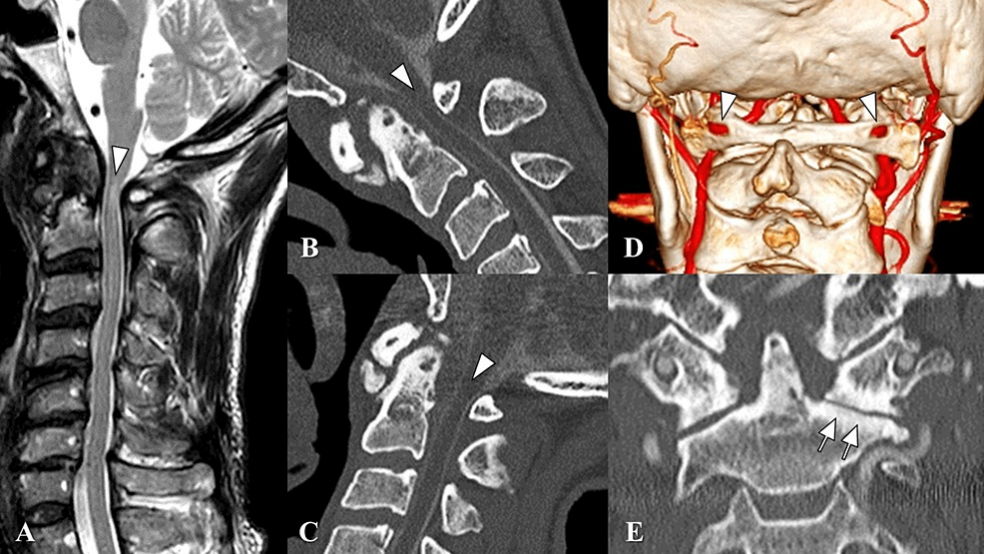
Preoperative images. A. T2-weighted magnetic resonance imaging (MRI) revealed severe spinal cord compression with a high signal change at the C1 spinal level (arrowhead). B and C. CT myelography showed that spinal cord compression was exacerbated during flexion (B; arrowhead) and released during extension (C; arrowhead). D. Three-dimensional CT angiography revealed that bilateral vertebral arteries (VA) ran through the ponticulus postics (arrowheads). E. Osteoarthritis at the left C1/2 facet joint was further observed (arrows) in the coronal CT scan.
